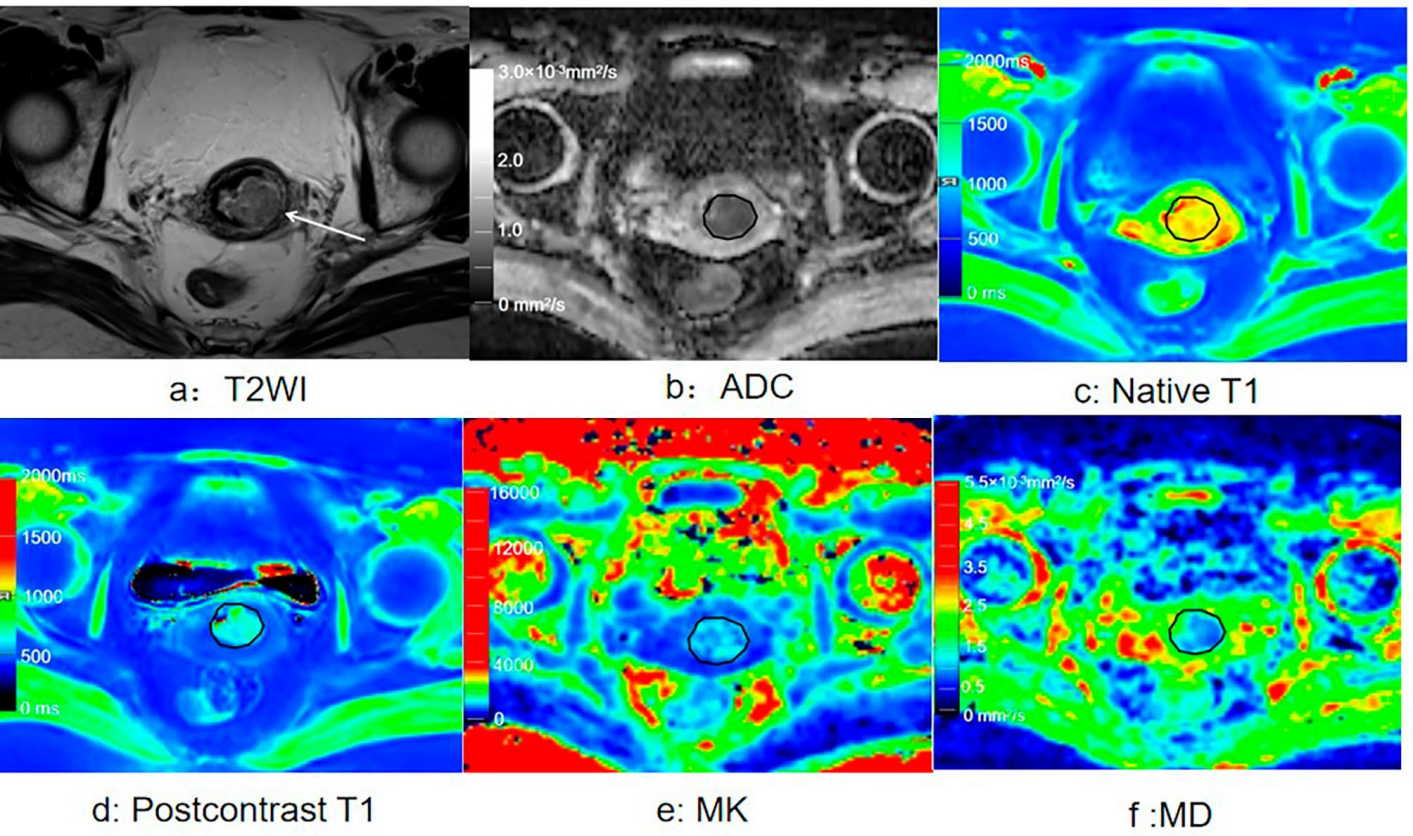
cervical adenocarcinoma (stage IB, low-grade). (a) Axial T2-weighted image shows a mass with a slight high-intensity signal in the cervix. (b) The mass showed low intensity on the ADC map. (c) Native T1 map. (d) Postcontrast T1 map. (e) MK map. (f) MD map
